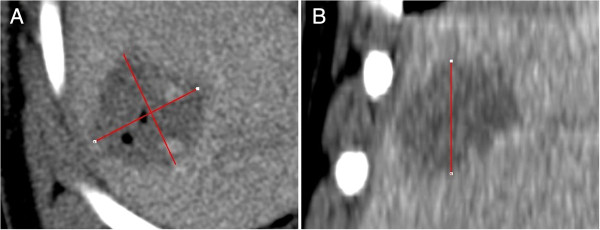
Technique B (Standard CT 3d Analysis) - Image Example. A, B axial image plane (A) as well as coronal image plane (B) – IRE Protocol 1. Note: in the axial image plane, the absolute longest diameter of the treatment zone was measured, and perpendicular to this parameter, the longest diameter of the treatment zone was determined. In the coronal image plane, the longest craniocaudal diameter of the treatment zone was determined. These three diameters were ordered by size and defined as long, intermediate and short diameter. The diameter of the largest inscribed sphere within the treatment zone was defined equal to the short diameter. The barycenter offset is not assessable applying this approach.
